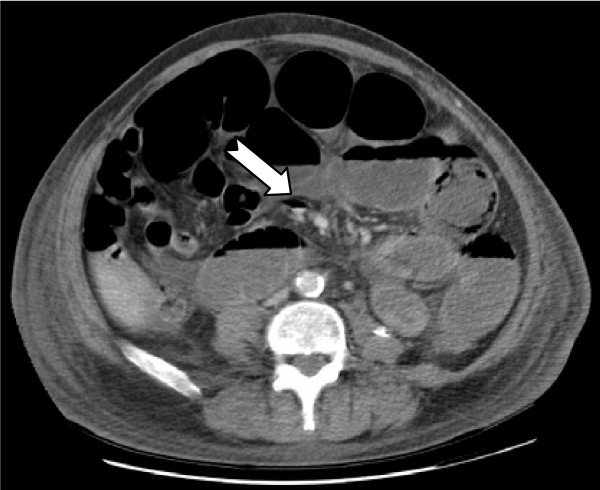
CT scan showing multiple dilated loops of small bowel with free fluid and air seen on the mesenteric border of the mid jejunum (arrow) suggesting perforation of the small bowel.
